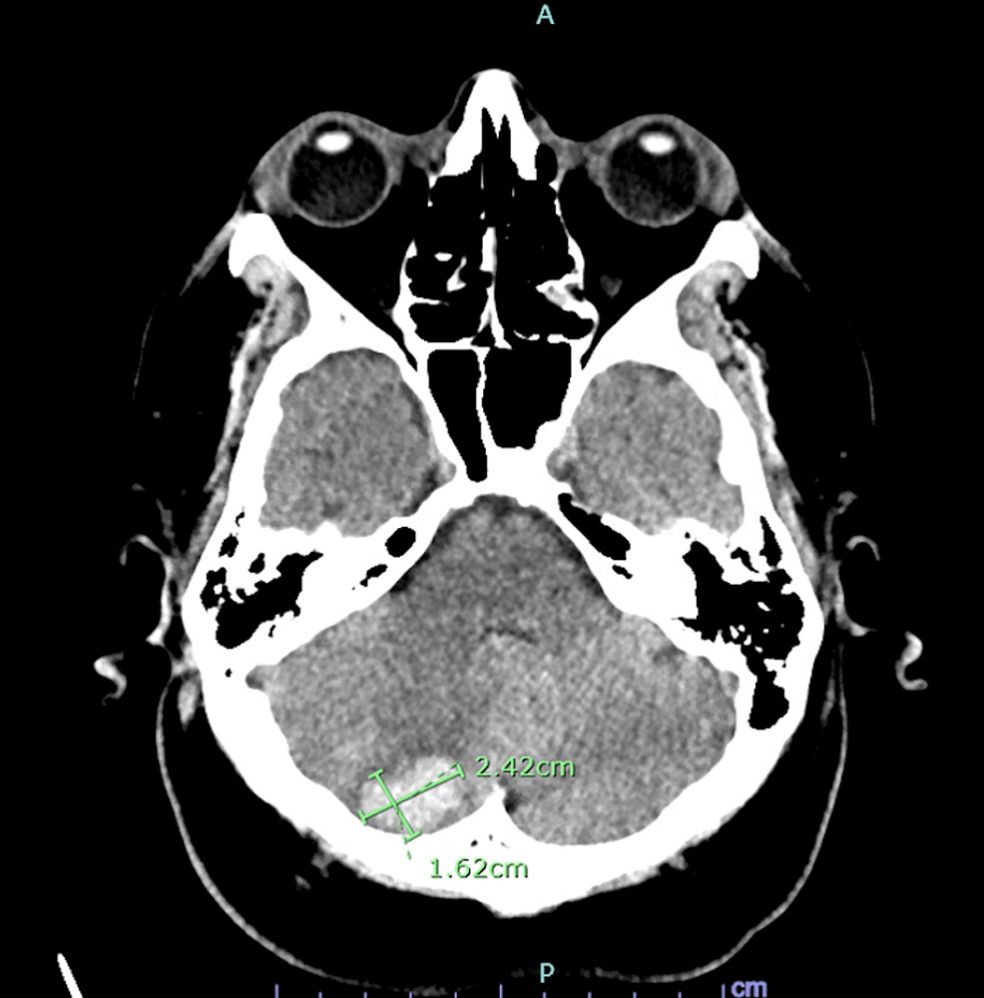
CT of the head without contrast. Moderate-sized right cerebellar parenchymal hematoma measuring 2.42 × 1.62 cm in transaxial dimensions. There is mild surrounding edema. Slight distortion of the adjacent fourth ventricle can be seen.
